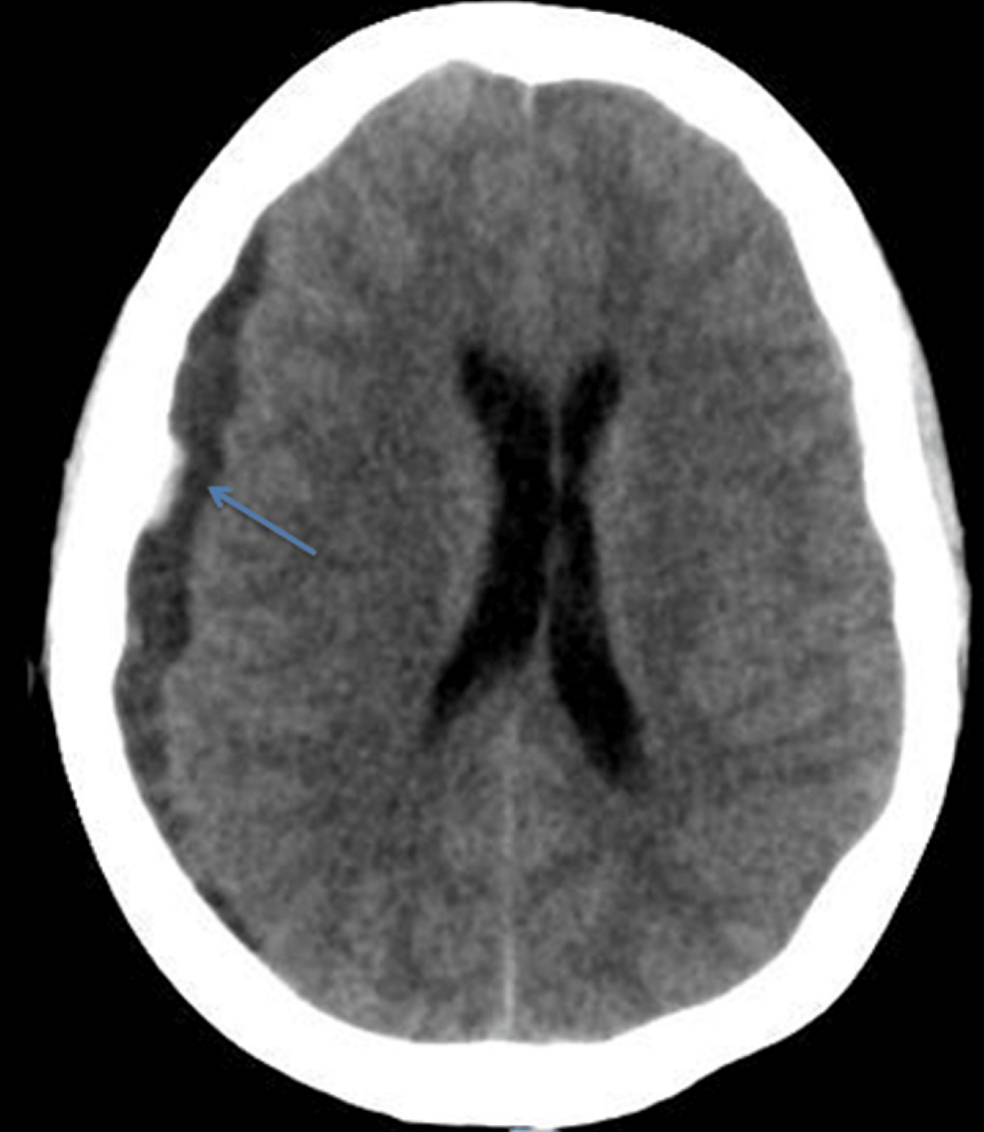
Acute on top of chronic subdural hematoma. Non-contrast CT scan (transverse view) showing chronic (hypodense) right-sided subdural hematoma (blue arrow) with a minimal hyperdensity.
